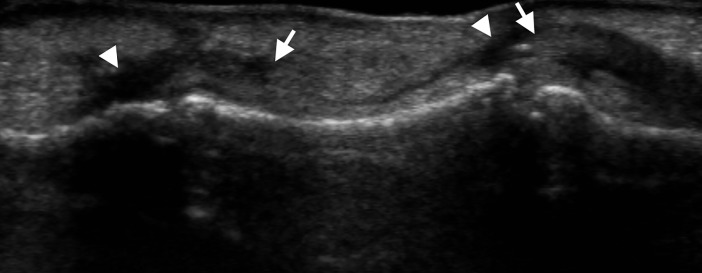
A 49-year-old woman with a flexor digitorum profundus tendon rupture of the ring finger. Ultrasound image of longitudinal scan shows discontinuity of the tendon with retraction of the ruptured tendon (arrows) at the middle phalangeal level. Anechoic lesions are seen around the ruptured tendon stumps and within the tendon sheath (arrowheads).
